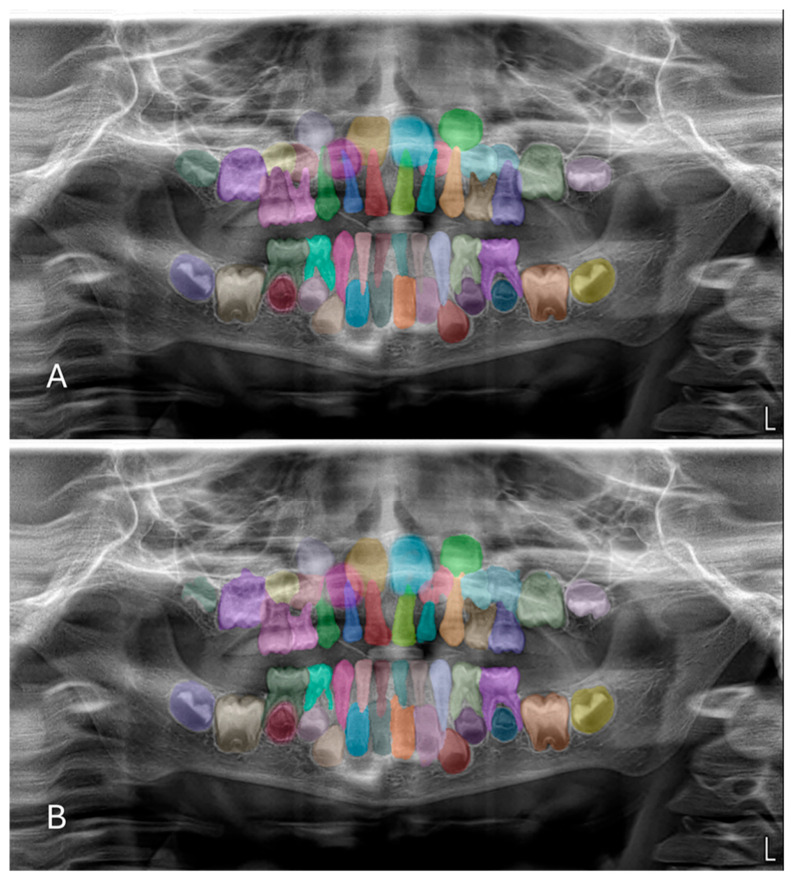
Example images demonstrating the performance for segmentation of mixed dentition on panoramic radiographs between manual annotation by researchers and U-Net based artificial intelligence (AI)-predicted label masks. (A) Manual segmentation performed by clinicians. (B) Automatic segmentation by the model. In the segmentation figures, each tooth is represented with a distinct colour to visually differentiate regions; however, the model treated all teeth as unified segmentation classes during training.
